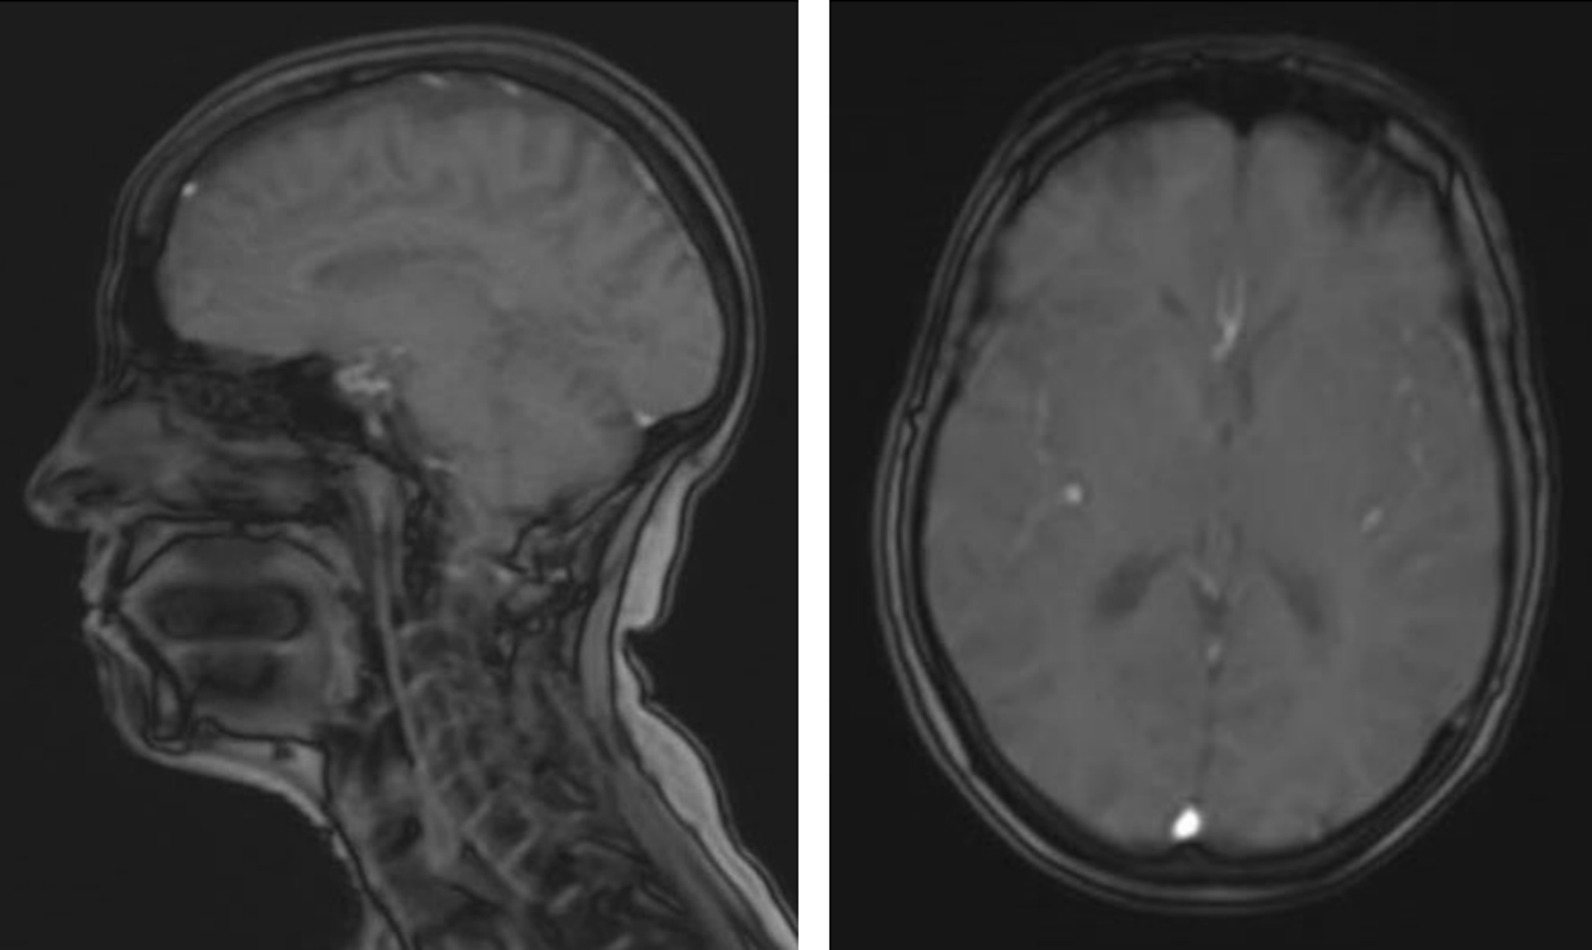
Magnetic resonance imaging findings
